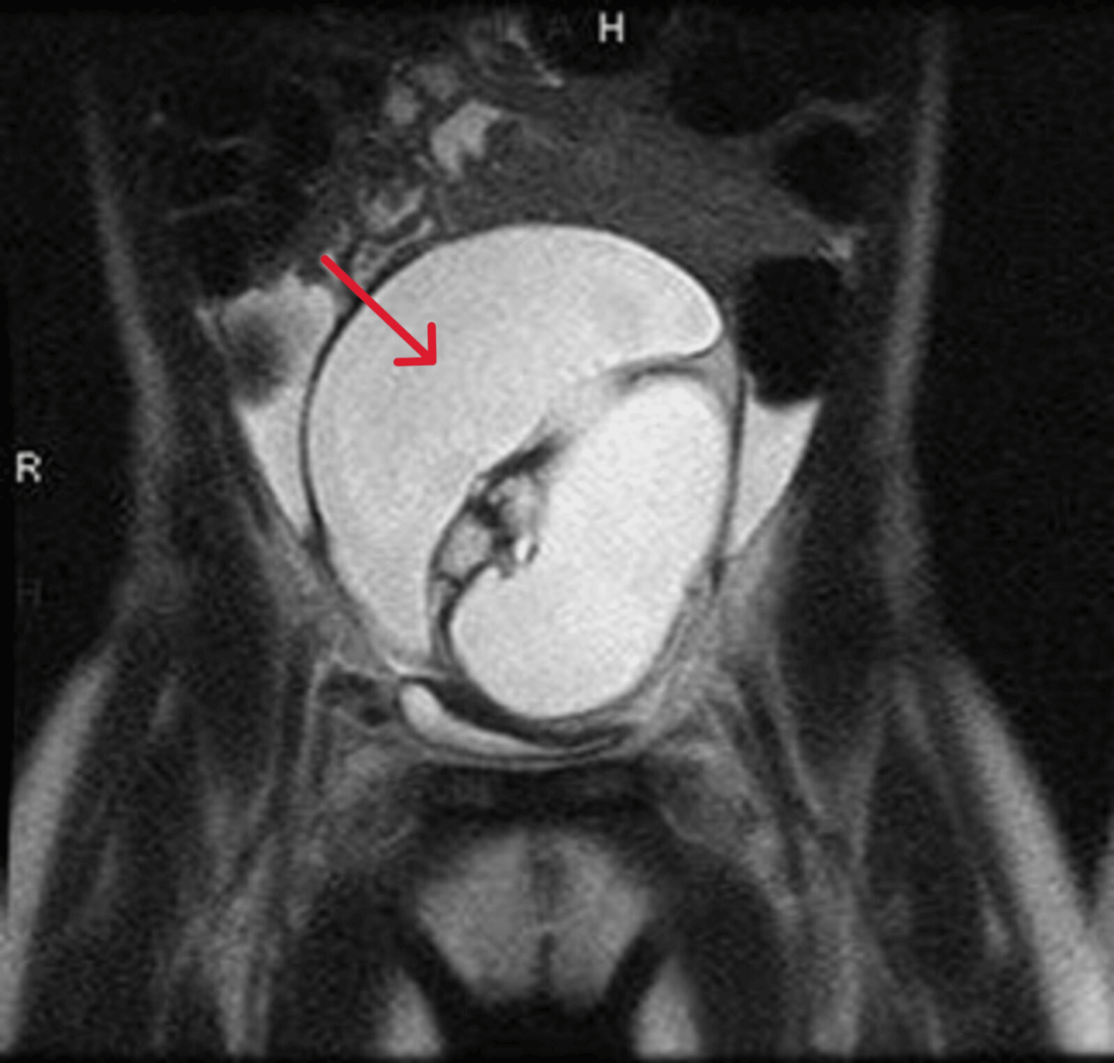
MRI report revealing a large complex cystic lesion in the pelvis, likely a mature cystic teratoma, measuring about 5.7×7.1×8.9 cm (arrow) The right ovary is not visualized, while the left ovary contains normal follicles. The uterus appears normal, and there is a small amount of free fluid noted. No lymphadenopathy or bowel abnormalities are present.
